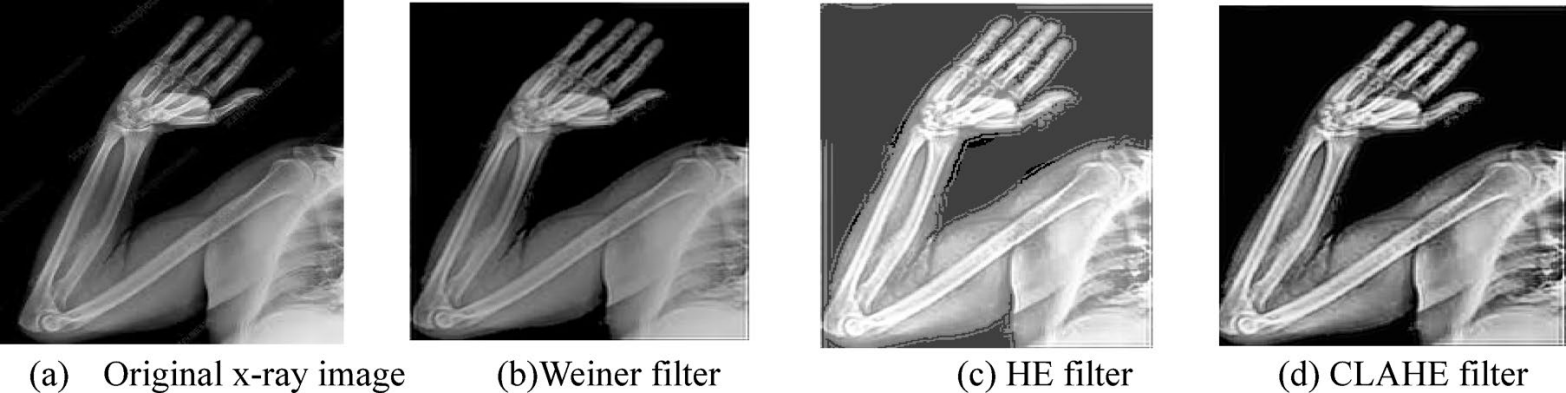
Examples of the outcomes of the three methods (Weiner, HE, and CLAHE filter) for enhancing X-ray image contrast.
